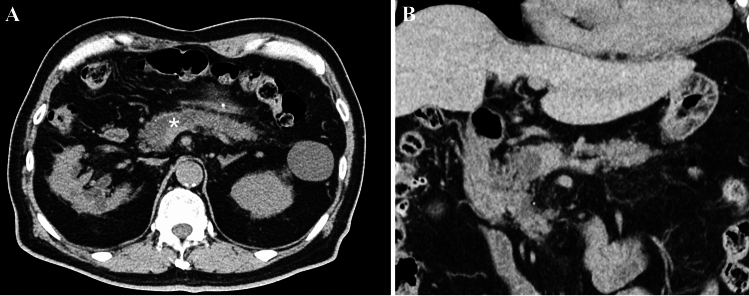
Axial (A) and coronal (B) images of the plain abdominal computed tomography (CT) findings. CT showed main pancreatic duct dilation (asterisk), and the pancreatic parenchyma showed mild atrophy in the body and tail, but the pancreatic mass was difficult to confirm
